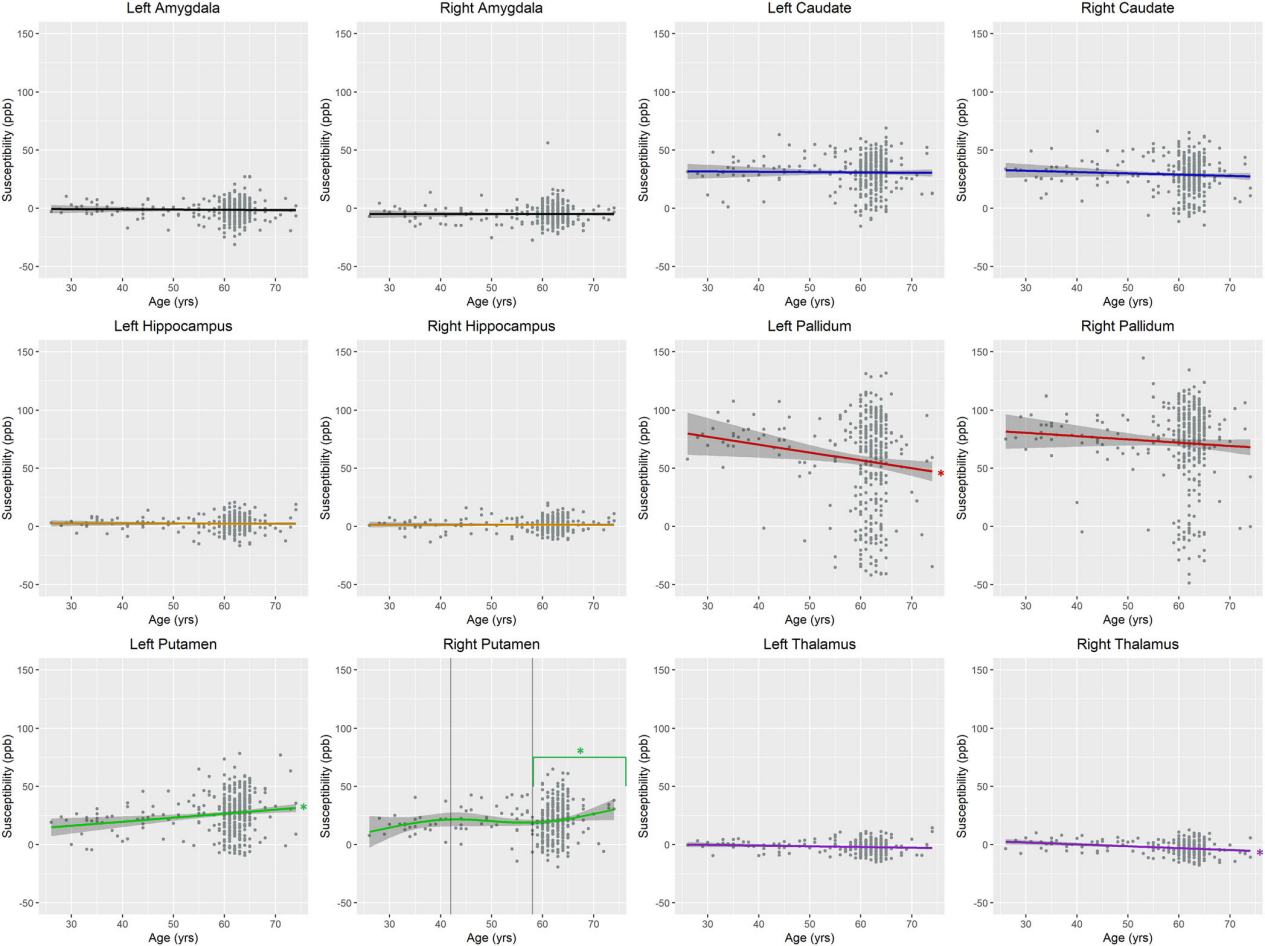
Relationships between iron content in grey matter regions as determined by quantitative susceptibility mapping on MRI and age at time of scan. * Indicates associations that were statistically significant.
